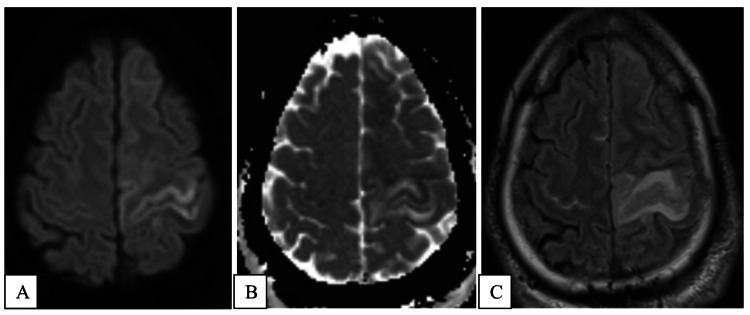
MRI sequences showing restricted diffusion in precentral gyrus. (A) DWI, (B) ADC, and (C) T2 Flair sequences showing restricted diffusion/hyperintense signal in a gyriform pattern spanning the precentral gyrus. DWI: diffusion-weighted imaging; ADC: apparent diffusion coefficient; MRI: magnetic resonance imaging.
